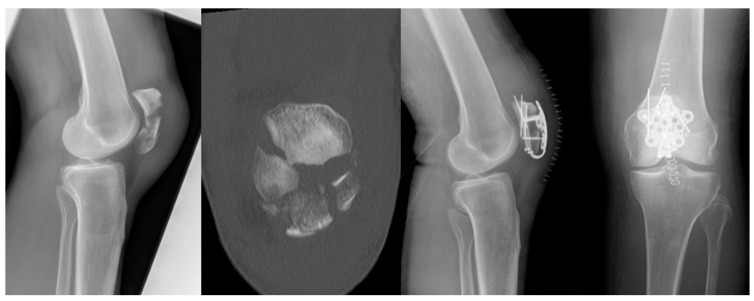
Radiographic and CT images of a 49-year-old female patient with a comminuted PF. Shown is an example of surgical treatment using patellar plate fixation with additional screw and K-wire osteosynthesis.
